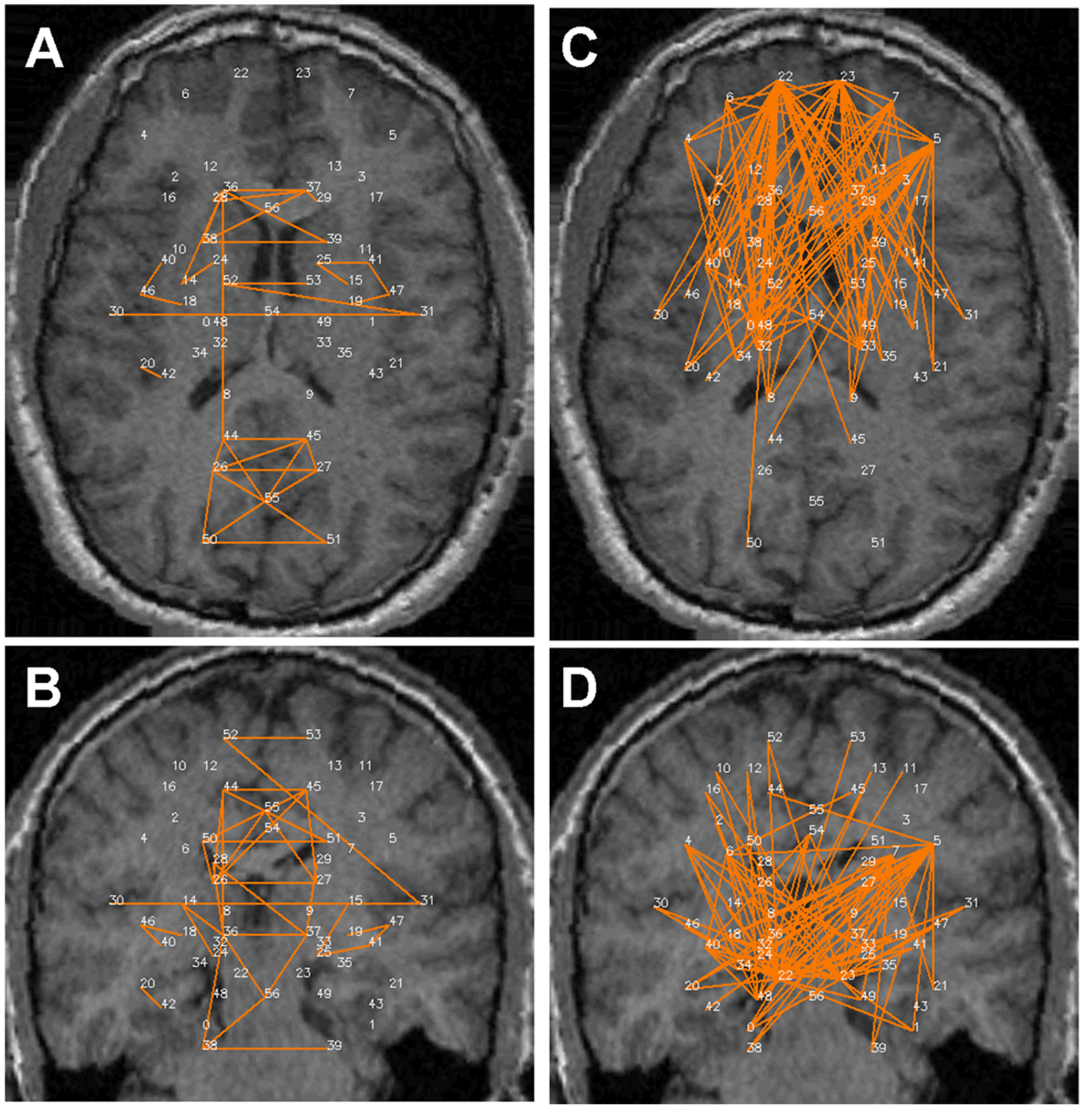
Significant connections within the 57 predefined human regions for the –S-G analysis. Significant connections are presented as 2D projections on top of T1-weighted coronal and axial MRI images. ROIs are annotated using numbers provided in Table 1. *A & B*. Positive connections. *C & D*. Negative connections.
